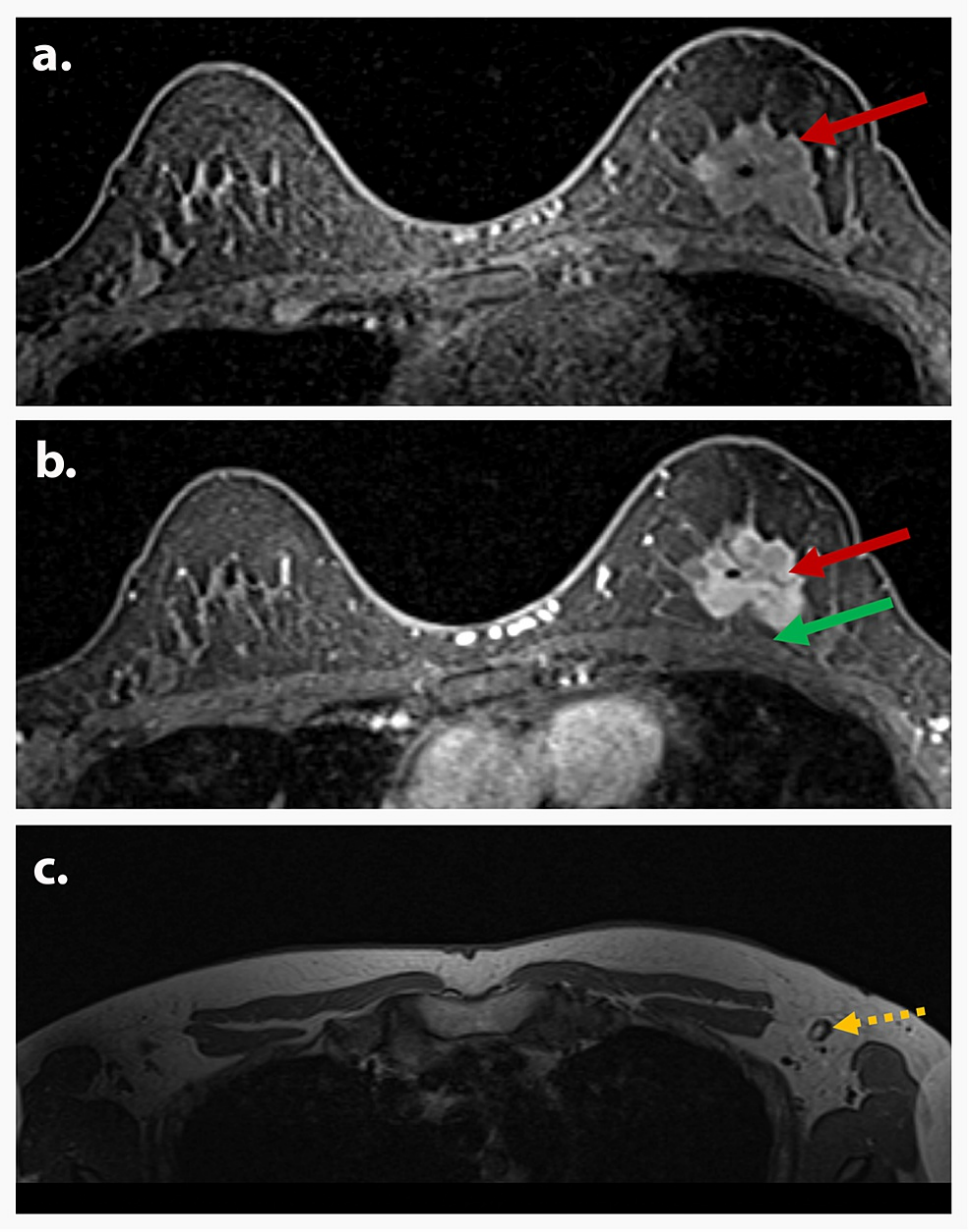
AJCC TNM stage IIA (T2N0M0) A 54-year-old presented with a left breast mass, which on evaluation with a diagnostic mammogram, ultrasound, and subsequent biopsy was proven to be invasive ductal carcinoma. Axial postcontrast T1-weighted subtraction MR image (a) shows an irregular heterogeneously enhancing posterior mass (red arrow) measuring 3.3 x 2.6 x 3.3 cm with spiculated margins in the upper central left breast, 12:00 position 3.9 cm from the nipple, with biopsy clip. Axial postcontrast T1-weighted MR image (b) demonstrates the irregular heterogeneously enhancing mass (red arrow) again with spiculated margins in the upper central left breast, with biopsy clip and without evidence of pectoral muscle infiltration (green arrow). Axial pre-contrast T1-weighted MR image (c) shows normal/physiologic non-enlarged axillary nodes (yellow arrow). Findings are consistent with AJCC TNM stage IIA (T2N0M0). AJCC: American Joint Committee on Cancer; TNM: tumor, node, and metastasis; MR: magnetic resonance.
